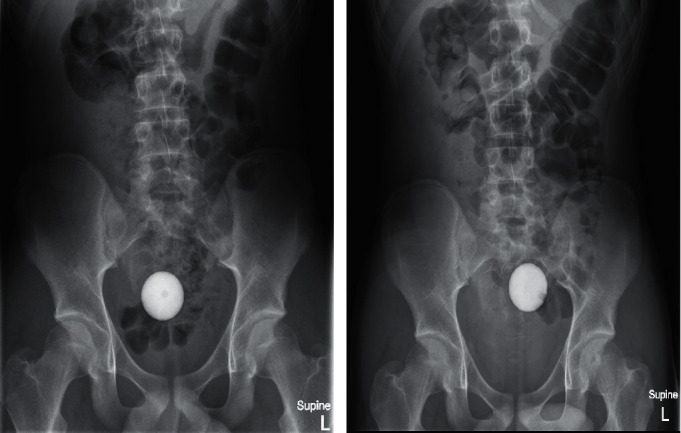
(a) Abdominal X-ray on admission demonstrating the golf ball within the upper rectum. (b) Abdominal X-ray on day 1 inpatient demonstrating proximal migration of the golf ball into the sigmoid colon.
